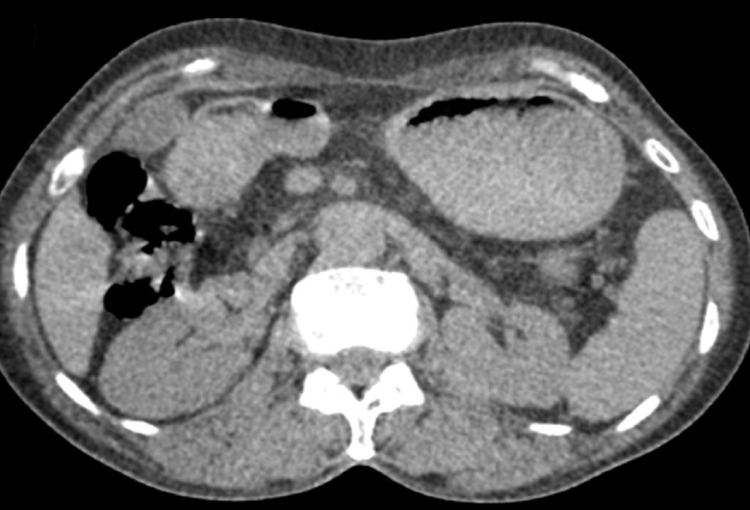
Control CT scan without injection of contrast medium (axial section): complete disappearance of the left ureteral hematoma and the homolateral perirenal infiltration without dilatation of the pyelocalic cavities.
